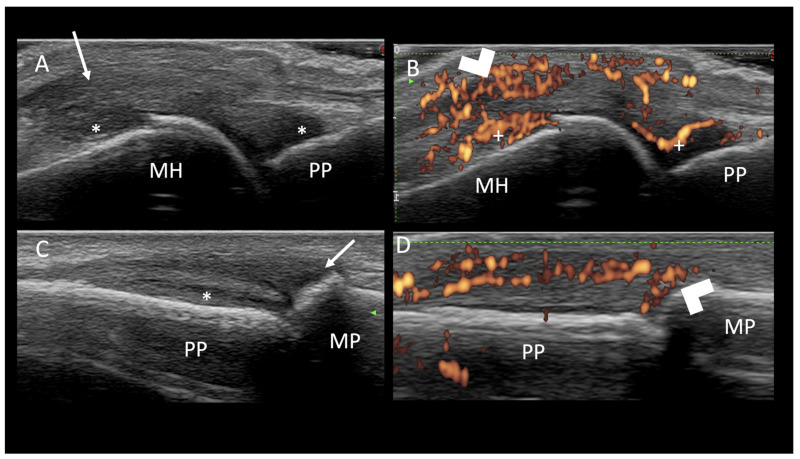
Longitudinal views of the metacarpophalangeal (A,B) and proximal interphalangeal joints (C,D) on ultrasound. (A) Synovial proliferation (∗) within the metacarpophalangeal joint and thickening, hypoechogenicity, and loss of fibrillary echotexture of the extensor tendon (arrow). (B) Intratendineous power Doppler signals (arrow head) in addition to the intrasynovial Doppler signals within the metacarpophalangeal joint (+). (C) Synovial proliferation of the proximal interphalangeal joint (∗) and thickening, hypoechogenicity, and loss of fibrillary echotexture of the extensor tendon insertion into the basis of the middle phalanx- enthesitis (arrow). (D) Entheseal power Doppler signals (arrow head).
